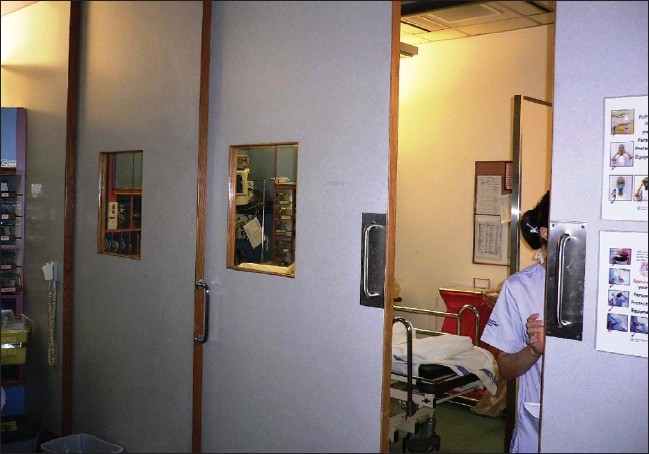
Negative pressure ventilation end cubicle in the resuscitation area with lead X-ray proof door partitions
